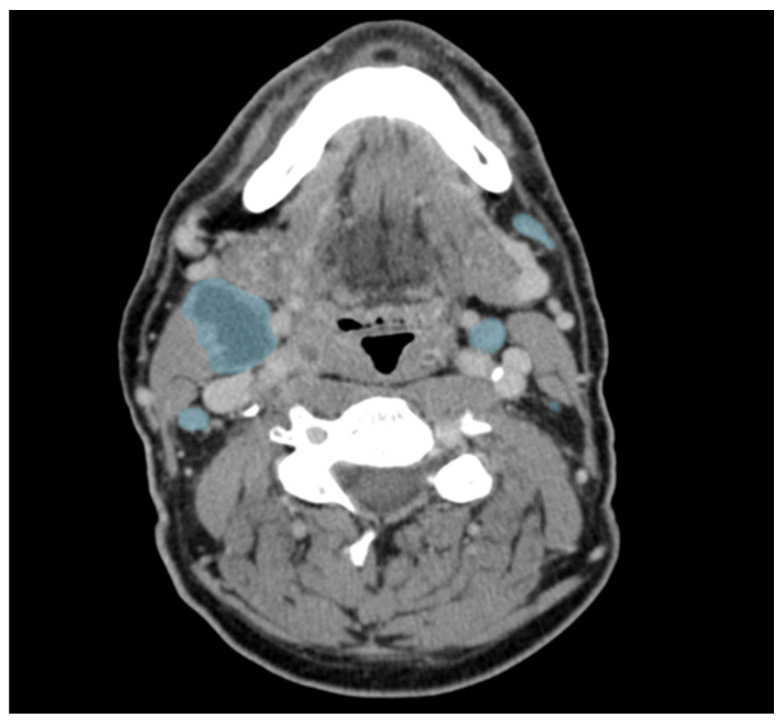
Example of manually corrected lymph node segmentations in an axial contrast-enhanced CT of a patient with head and neck cancer. Lymph nodes are marked in blue. Please note the central necrosis (more hypodense areas) of the large lymph node on the patient’s right.
